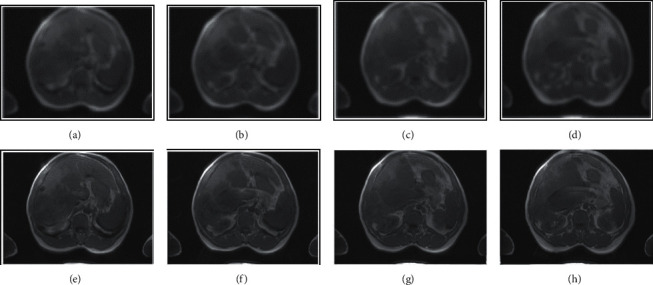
MRI images of a 55-year-old male patient with liver cancer. (a–d) *T*1, *T*2, *T*1*W*1, and *T*2*W*2 phase maps processed by traditional CNN MRI image segmentation algorithm; (e–h) *T*1, *T*2, *T*1*W*1, and *T*2*W*2 phase maps processed by the upgraded 3D CNN-based MRI image segmentation algorithm.
